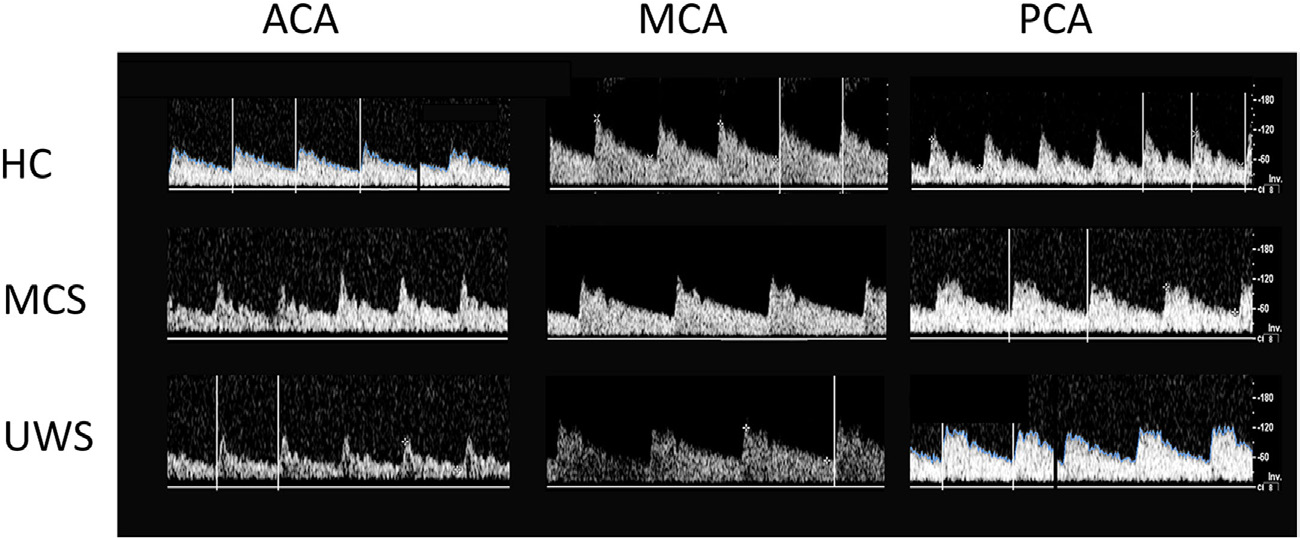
Shows the transcranial Doppler waveforms (on the right) from left middle (MCA), anterior (ACA), and posterior (PCA) cerebral arteries at rest in a representative subject for each group (HC, MCS, and UWS). Flow velocity (in centimeter per second, right vertical bar) are provided.
